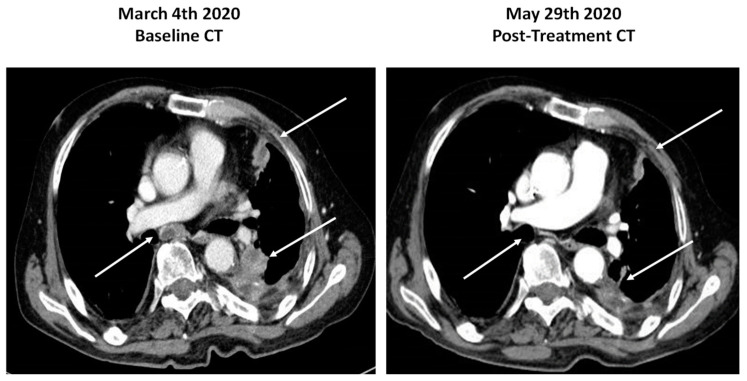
CT scans of the lung lesions before and after chemotherapy with carboplatin and pemetrexed. Arrows highlight the significant response to therapy of mediastinal, pleural, and lung lesions.
